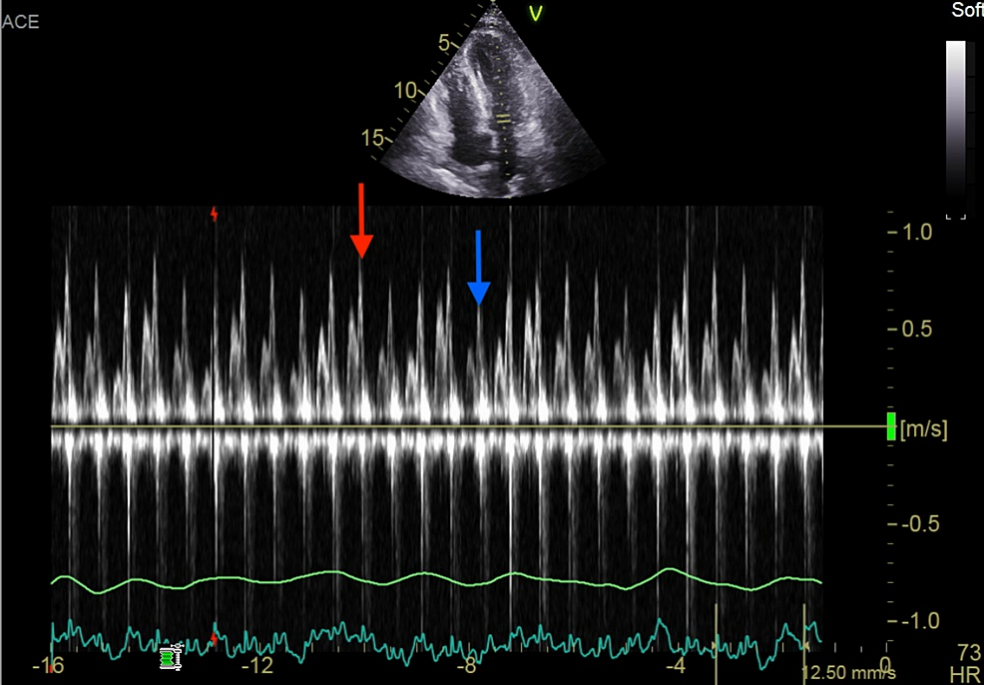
Echocardiogram Doppler view showing a variation of transvalvular flow velocity across the mitral valve The peak flow velocity during expiration is 0.9 m/s (red arrow) and 0.63 m/s during inspiration (blue arrow), which is a 30% decrease, a finding characteristic in cardiac tamponade.
